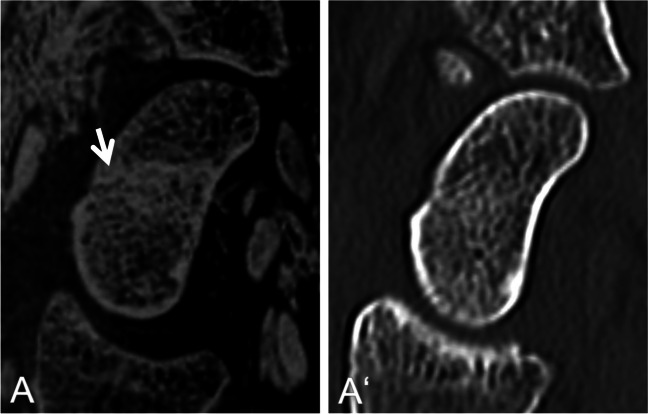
Twenty-six-year-old patient with a suspected fracture of the scaphoid (arrow). Multiplanar reconstruction in parasagittal plane (A, A‘) based on an inverted 3D T1GRE sequence (A) and a CT (A’). Fracture not clearly seen on CT. Time interval between CT and MRI 4 days. Note, the subtle fracture line with the adjacent oedema in A, rated as false positive
